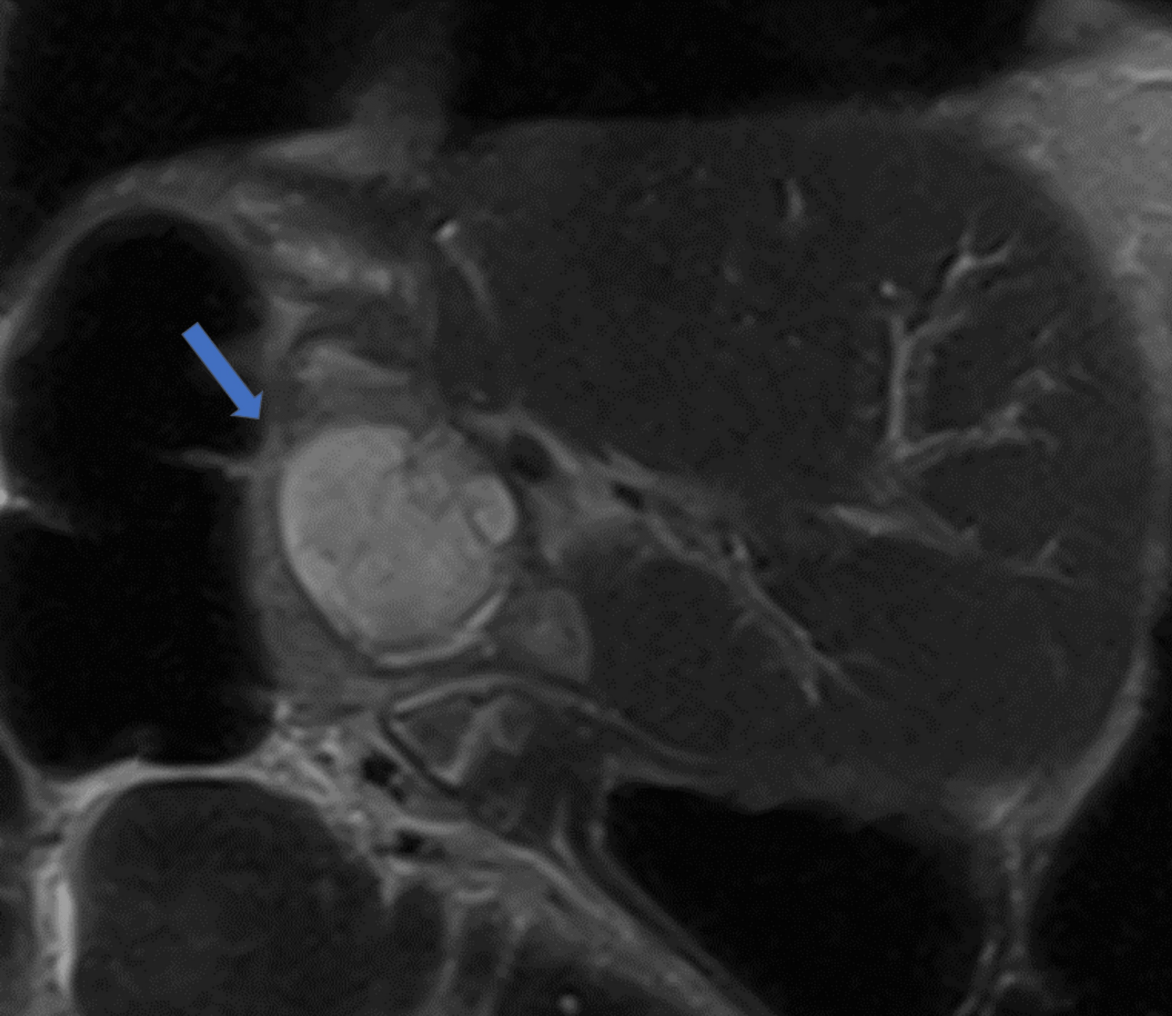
Postoperative MRI imaging MRI coronal view demonstrating the complex collection (arrow) causing pressure to the overlying hepatico-jejunostomy. MRI: magnetic resonance imaging
